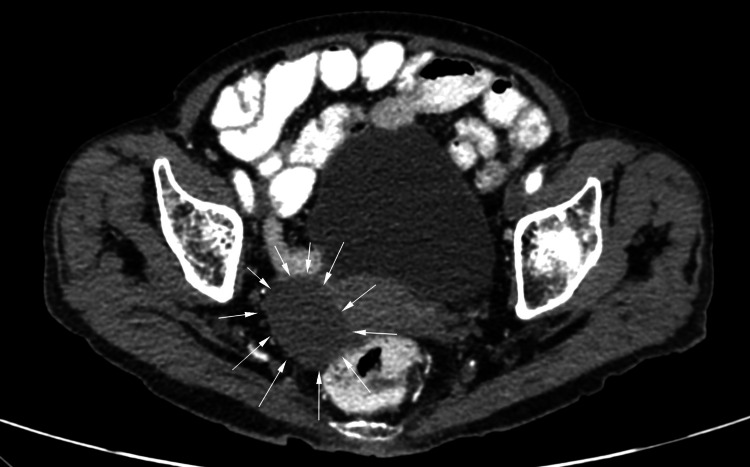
Abdominopelvic CT showing right ovarian cyst Well-defined cyst with regular borders and no local invasion
